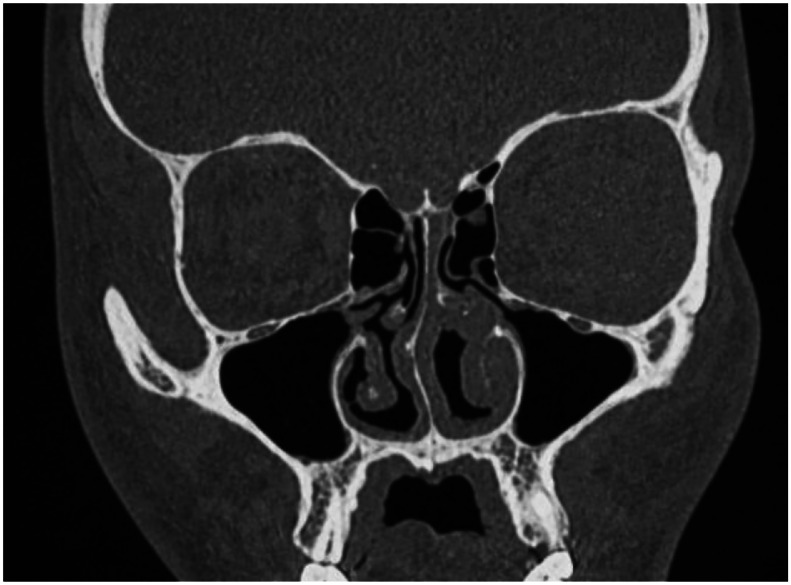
Non-contrast coronal CT scan (bone window) demonstrating a slight reduction in the size of the nasal septal perforation compared with the initial examination. No progression of osseous destruction is observed.
